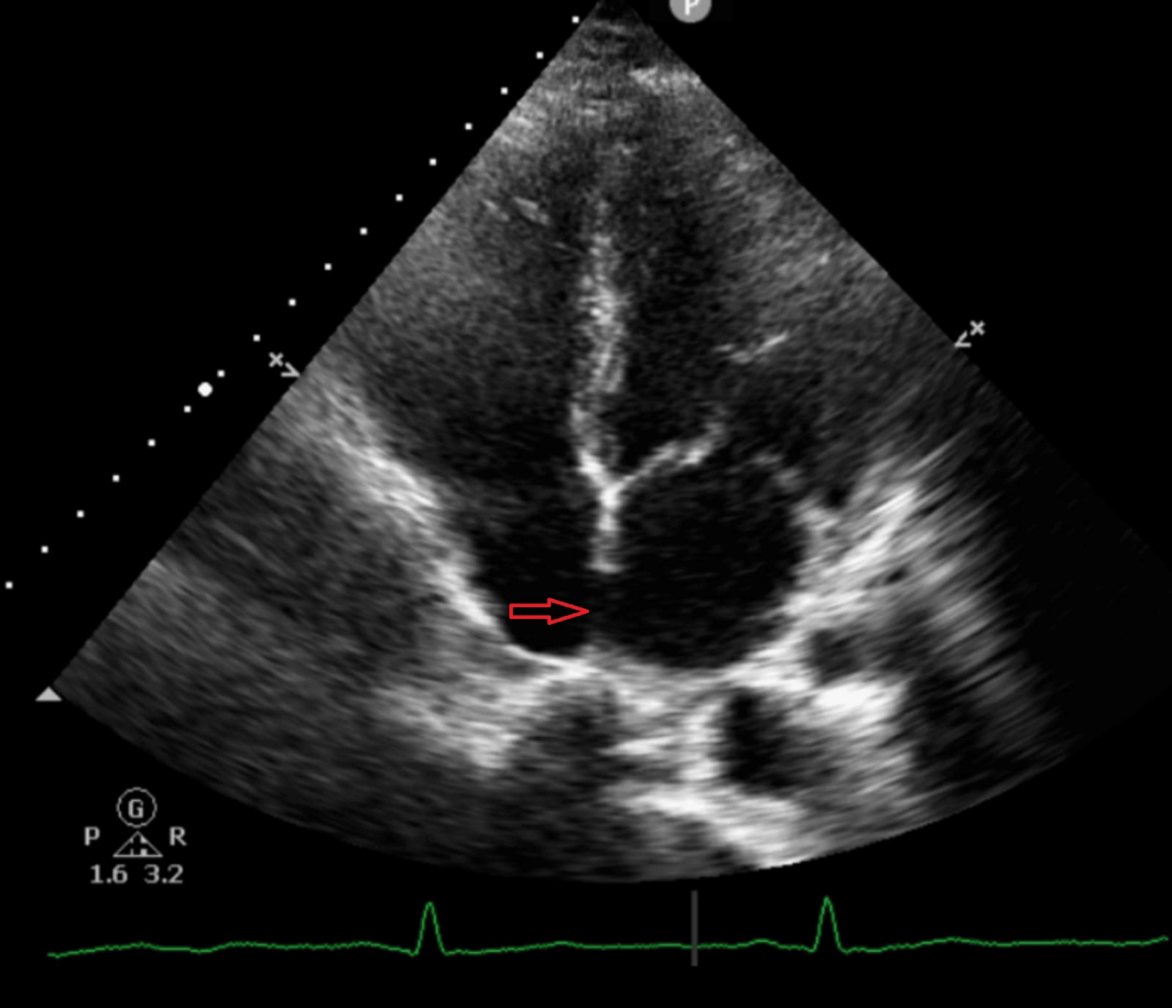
Echocardiogram (four chamber view) Transthoracic echocardiogram showing a patent foramen ovale in the interatrial septum.
